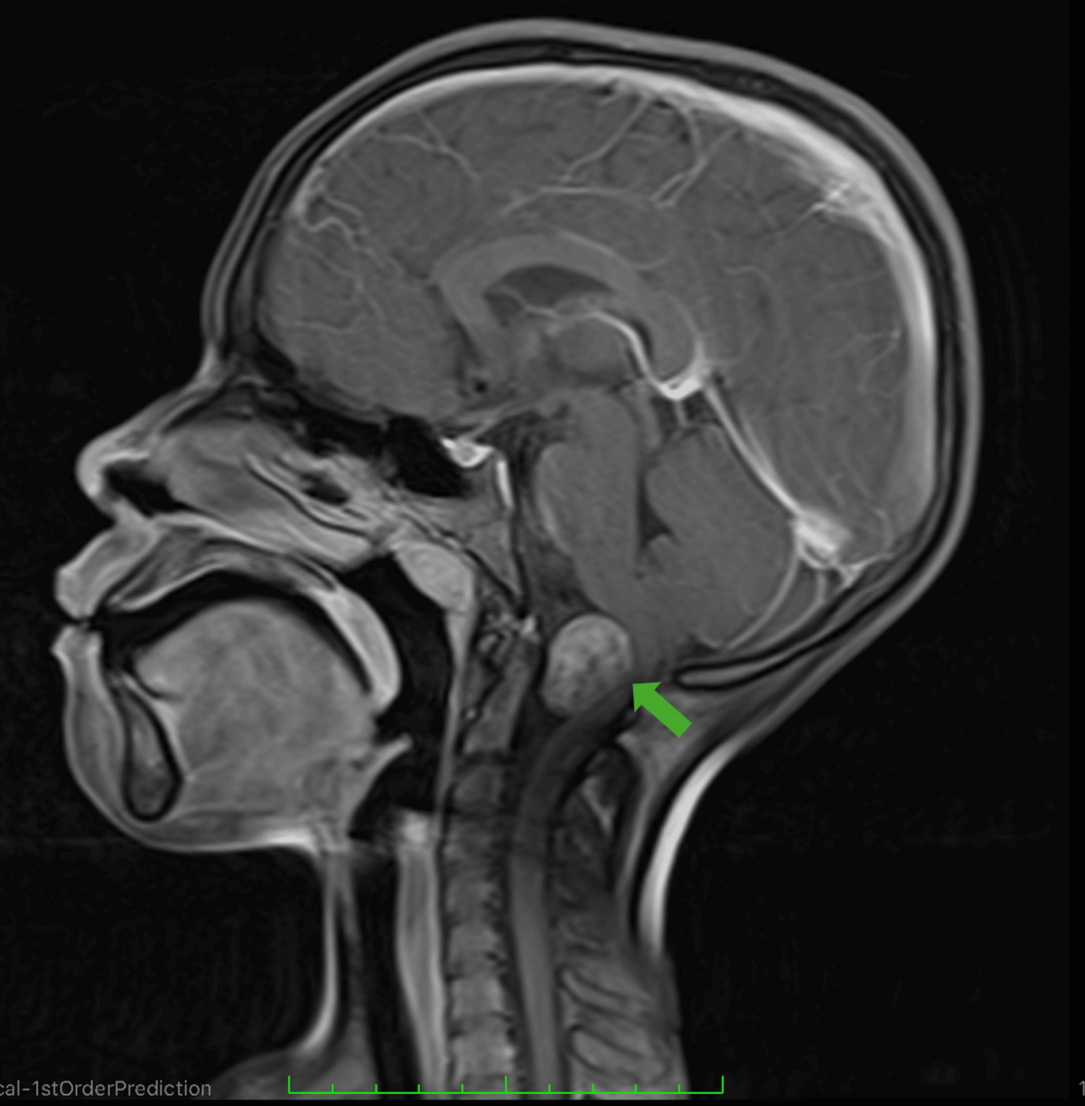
Preoperative neuroimaging. Contrast-enhanced magnetic resonance imaging (gadolinium) of the brain and craniocervical junction showed the lesion was hyperintense on T2 and had an oval-shaped image with avid and heterogeneous enhancement, measuring 2.5 × 2 × 2 cm in its longitudinal, anteroposterior, and transverse diameters (green arrow), and was displacing the medulla oblongata posteriorly.
